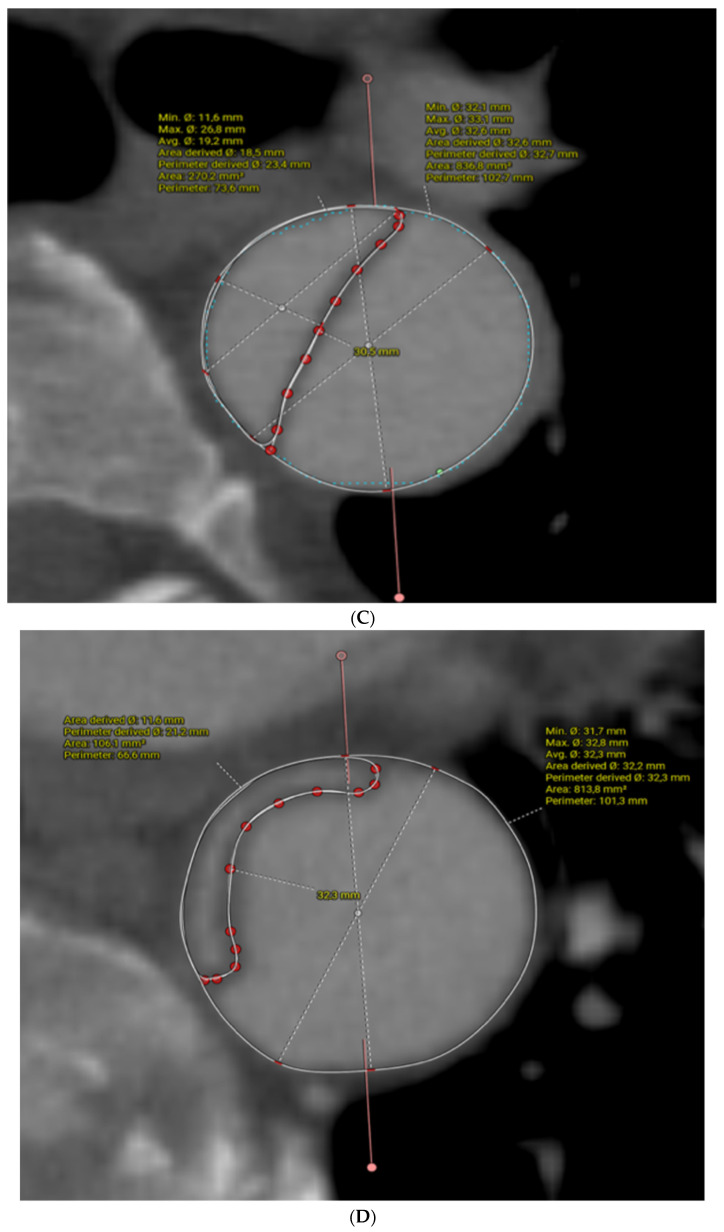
Pre-operative CT angiogram analysis for sizing a FET in acute type A aortic dissection, using centreline analysis and multiplanar reconstruction. Yellow line is the centreline and green marks the outer curve in (A). (B) is the vessel stretch display where the distal landing zone indicated by the arrow is estimated. (C,D) demonstrate the measurement of the dissection septum and aortic diameter. See full text for further explanation.
